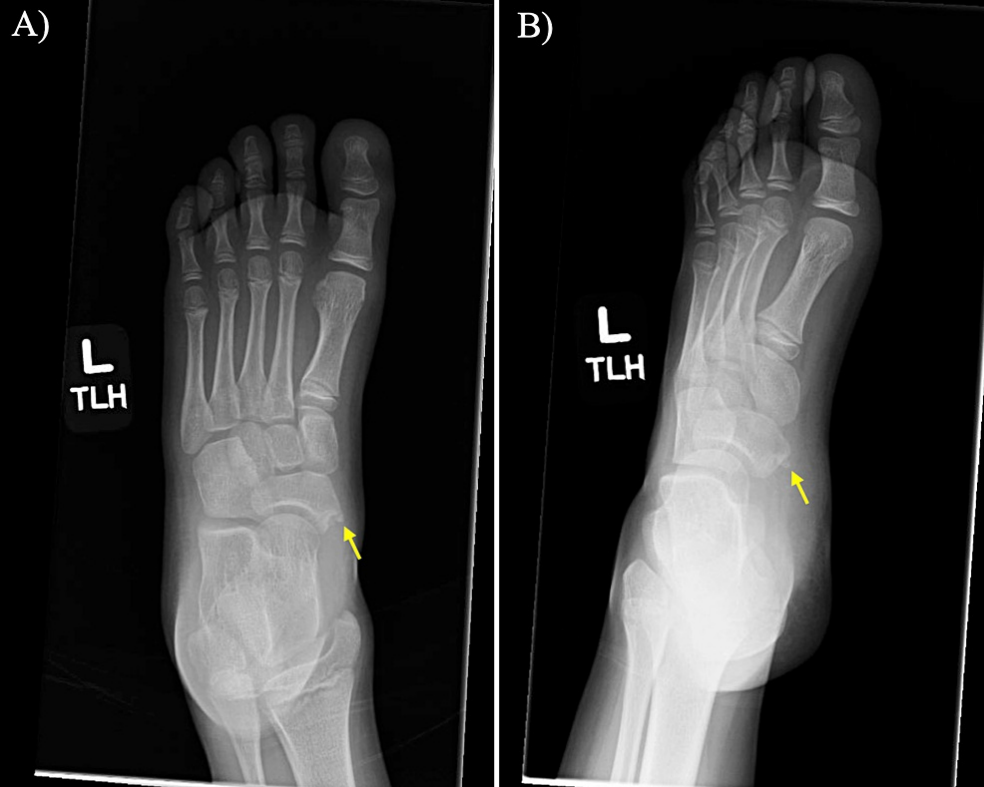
Preoperative foot and ankle radiographs A) Anterior-posterior (AP) view X-ray of the left foot demonstrating avulsed ossification center (yellow arrow) and possible second ossification center at the time of initial presentation; B) Oblique view X-ray of the left foot at the time of initial injury demonstrating fractured navicular ossification center (yellow arrow).
